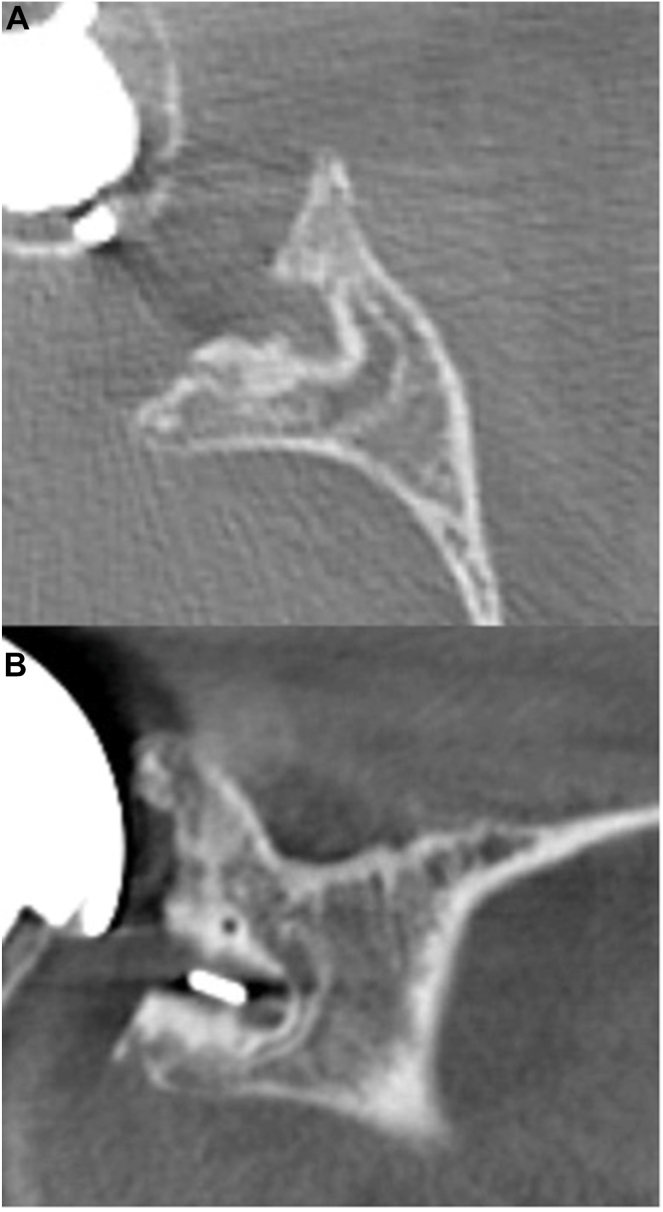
Two-dimesional computed tomography (CT) images of the contained glenoid defect. (A) Axial and (B) coronal images.
